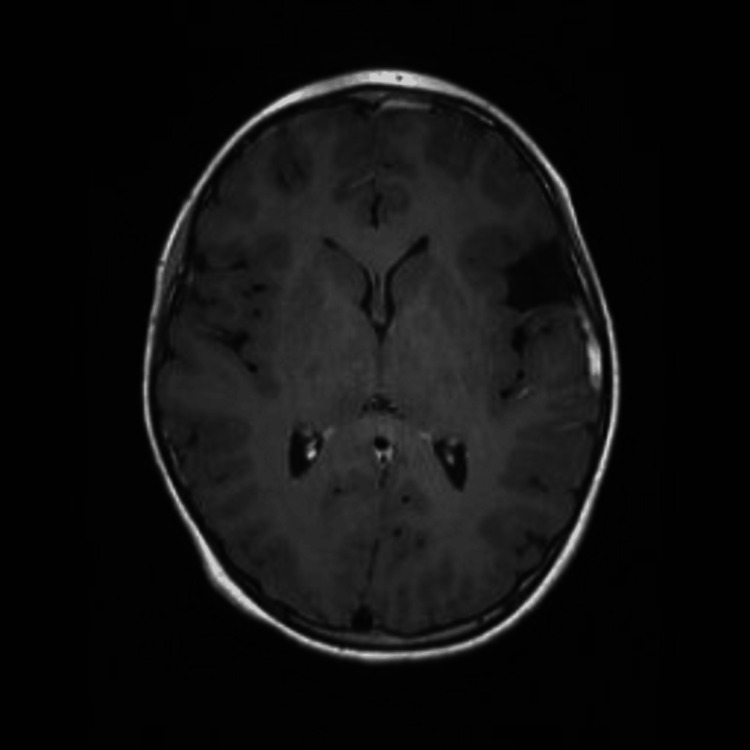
Axial, non-contrast MRI three weeks after hospital admission reveals resolving epidural abscess.
